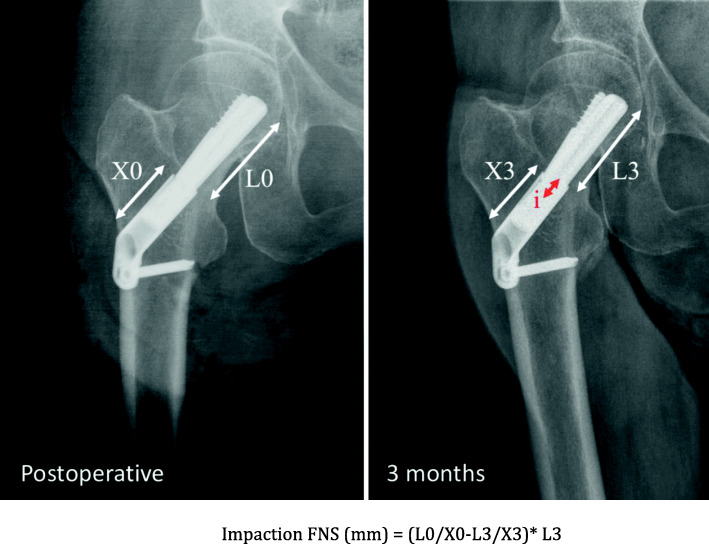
Calculation of impaction with FNS implant. Postoperative and 3-month X-ray
